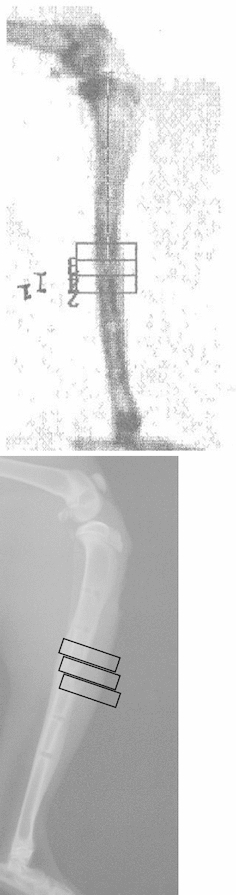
DXA image with corresponding radiograph
